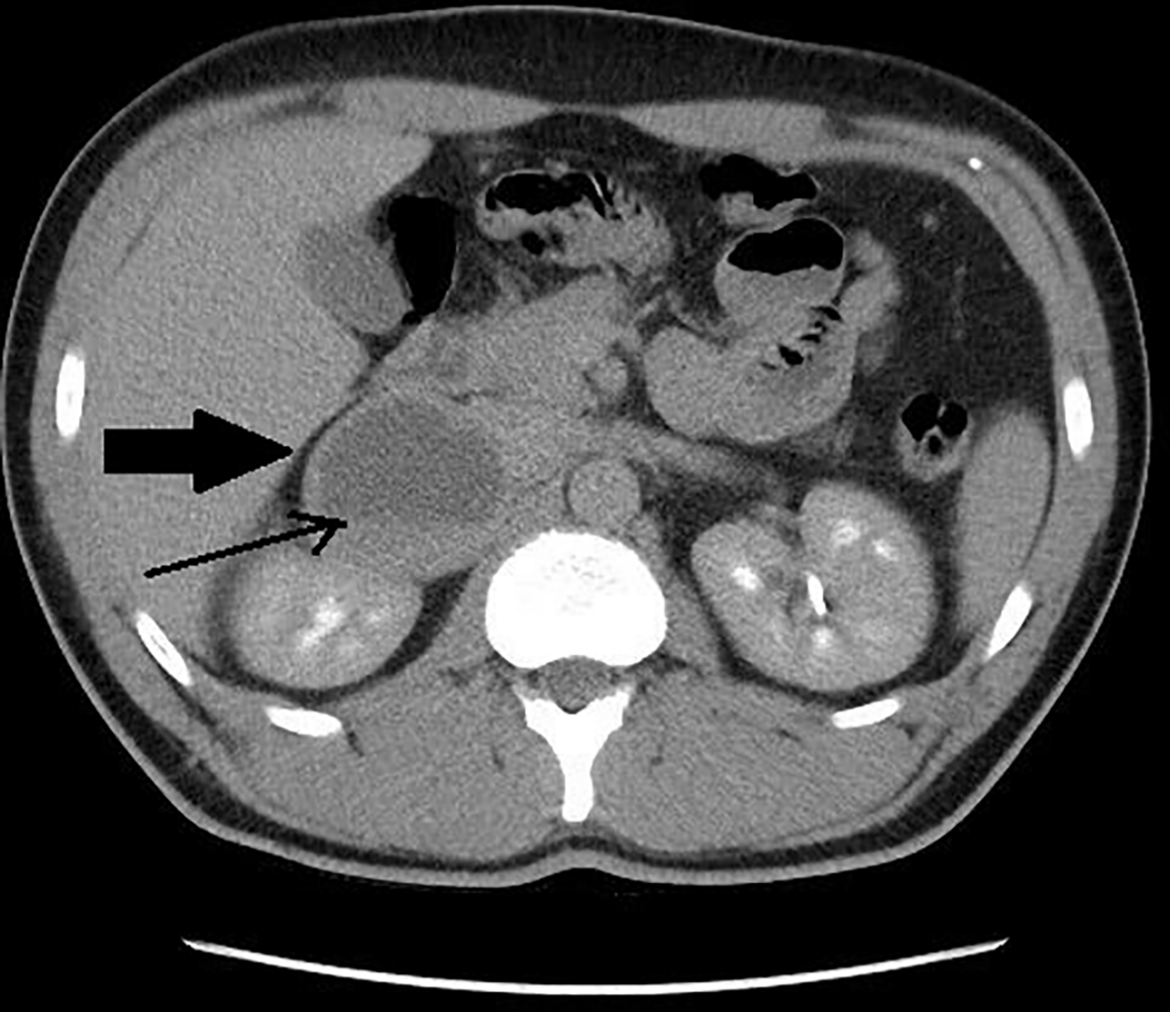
Patient number 7: CT of the abdomen, venous phase, axial image – right adrenal lesion with solid-cystic appearance (thick arrow), central area of fluid attenuation, with fluid-fluid level (thin arrow).
